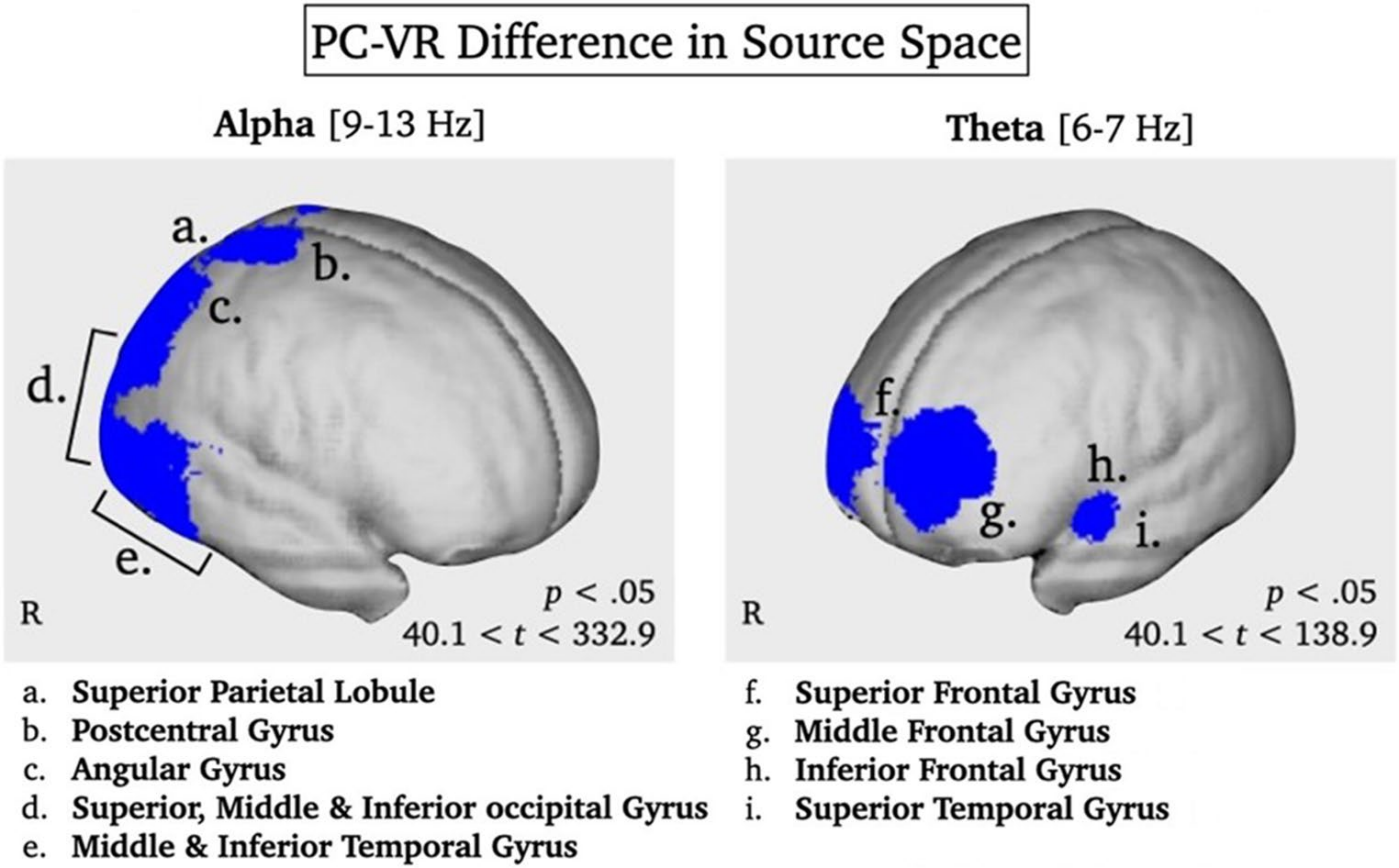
Statistically significant sources of the PC-VR difference of the mean iABR (9–13 Hz; 250–600 ms) and the mean iTBR (6–7 Hz; 300–650 ms) across stimulus types projected onto the surface of an average MRI template brain. Statistically significant differences in activity between modalities are marked in blue (*p* < .05, *t*_crit_ = 40.1)
